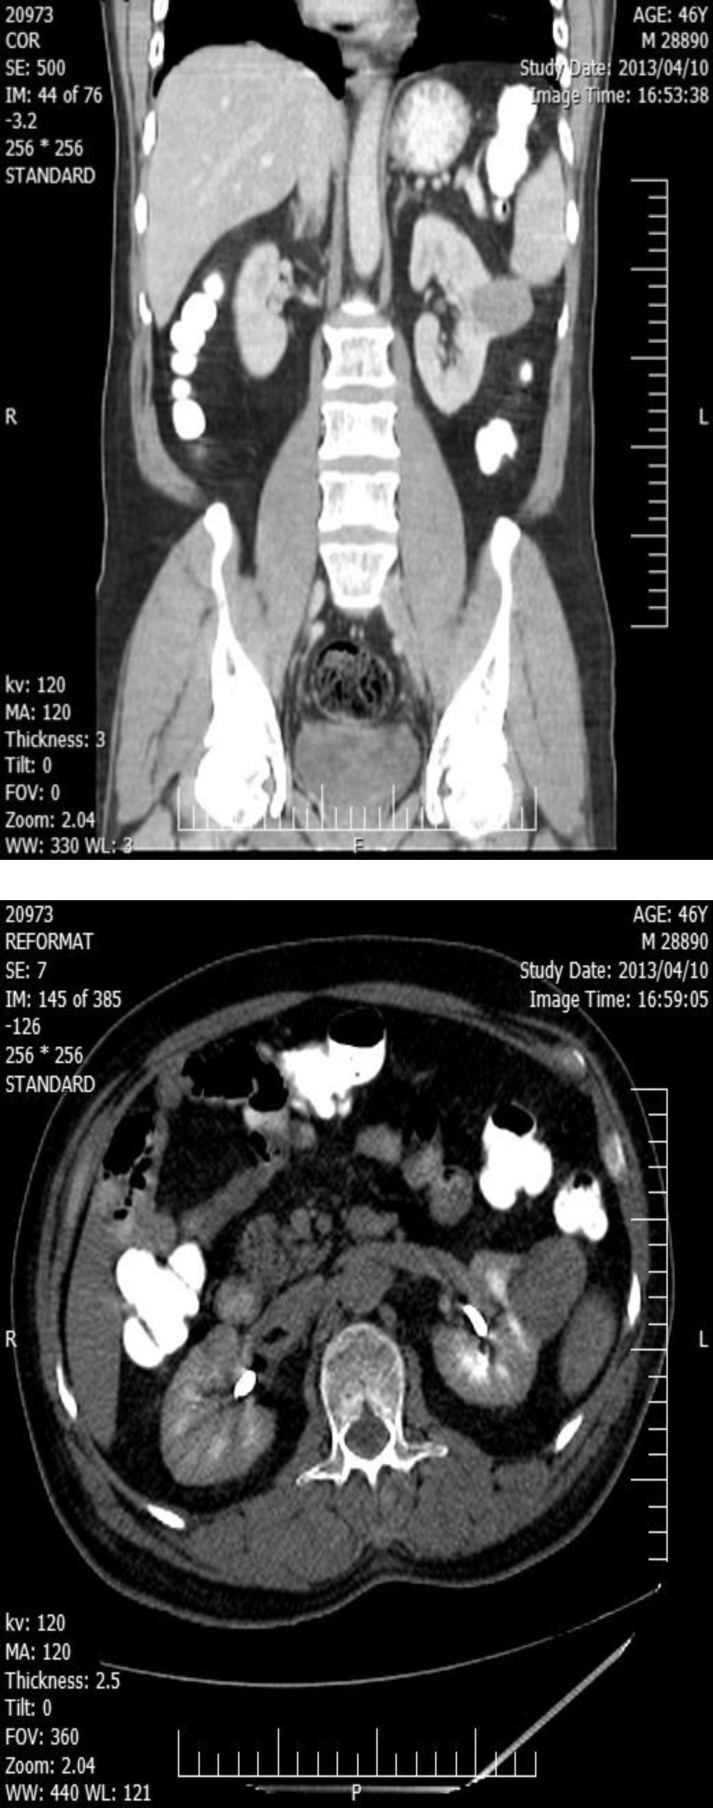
CT scan revealed a well-defined solid mass (34 x 46 x 36 mm) within the left kidney
